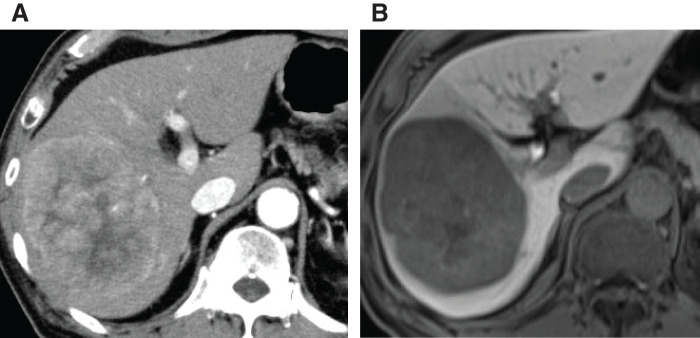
Abdominal contrast-enhanced CT (A) and MRI (B) findings before operation. (A) An enhanced mass measuring 97 × 78 mm in diameter is identified in the right lobe of the liver on arterial-phase imaging. (B) A large mass measuring 100 × 80 mm in diameter is observed in the right lobe of the liver, and the tumor boundary appears clear on the hepatobiliary phase image.
